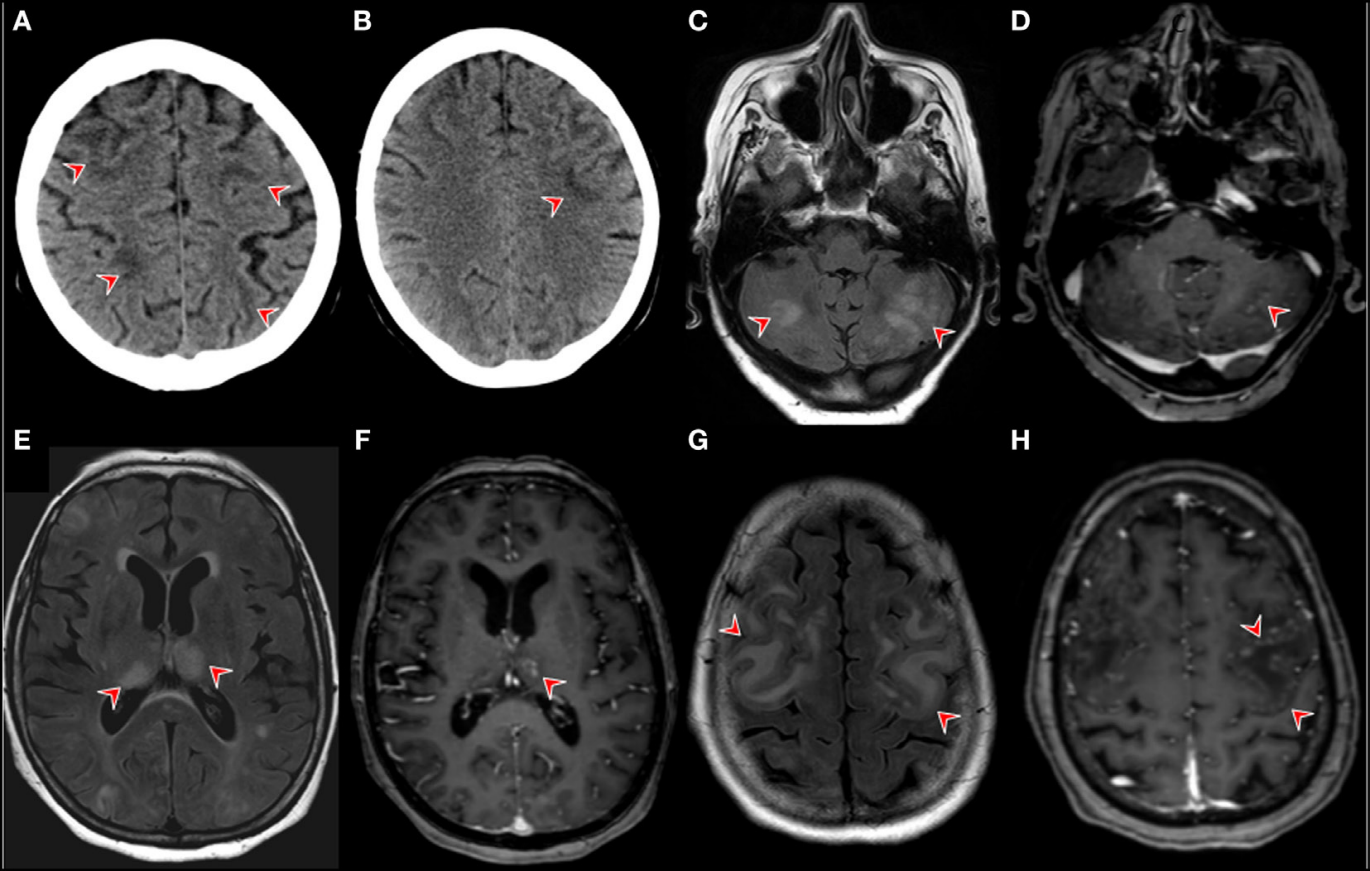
Neuroimaging. Brain CT in the postoperative course after the patient developed nausea, vomiting, and generalized weakness. The red arrows point at revealing wide-spread bilateral hypodensities in the subcortical white matter of the frontal, parietal, and occipital lobe (A,B). Brain MRI findings on day 14 of month 1 of the first nivolimab course. Fluid-attenuated inversion recovery (FLAIR) magnetic resonance imaging (MRI) showing multiple bilateral hyperintensities in gray cerebellar matter [(C), red arrows]. (D) T1-contrast enhanced images on the same level as image [(A) (red arrow)]. (D) MRI FLAIR images showing bilateral thalamic hyperintensities with corresponding T1-contrast enhancement left [(F), red arrow]. FLAIR MRI images showing confluent cortical hyperintensities (G) T1 contrast-enhancement showing pial gyriform pattern of enhancement [(H), red arrows].
